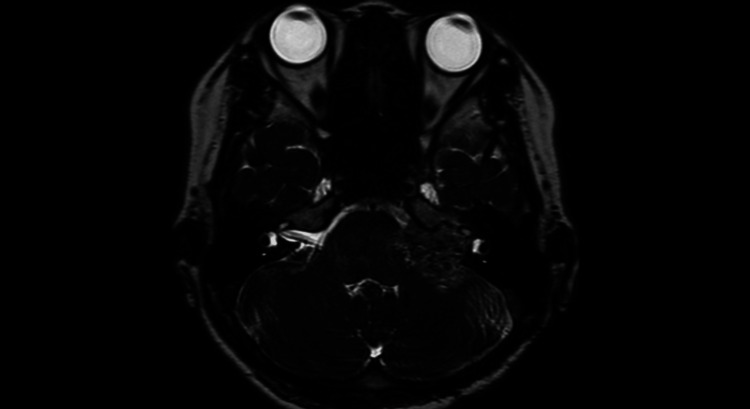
A 2.5 years postoperative MRI heavy T2-weighted image
